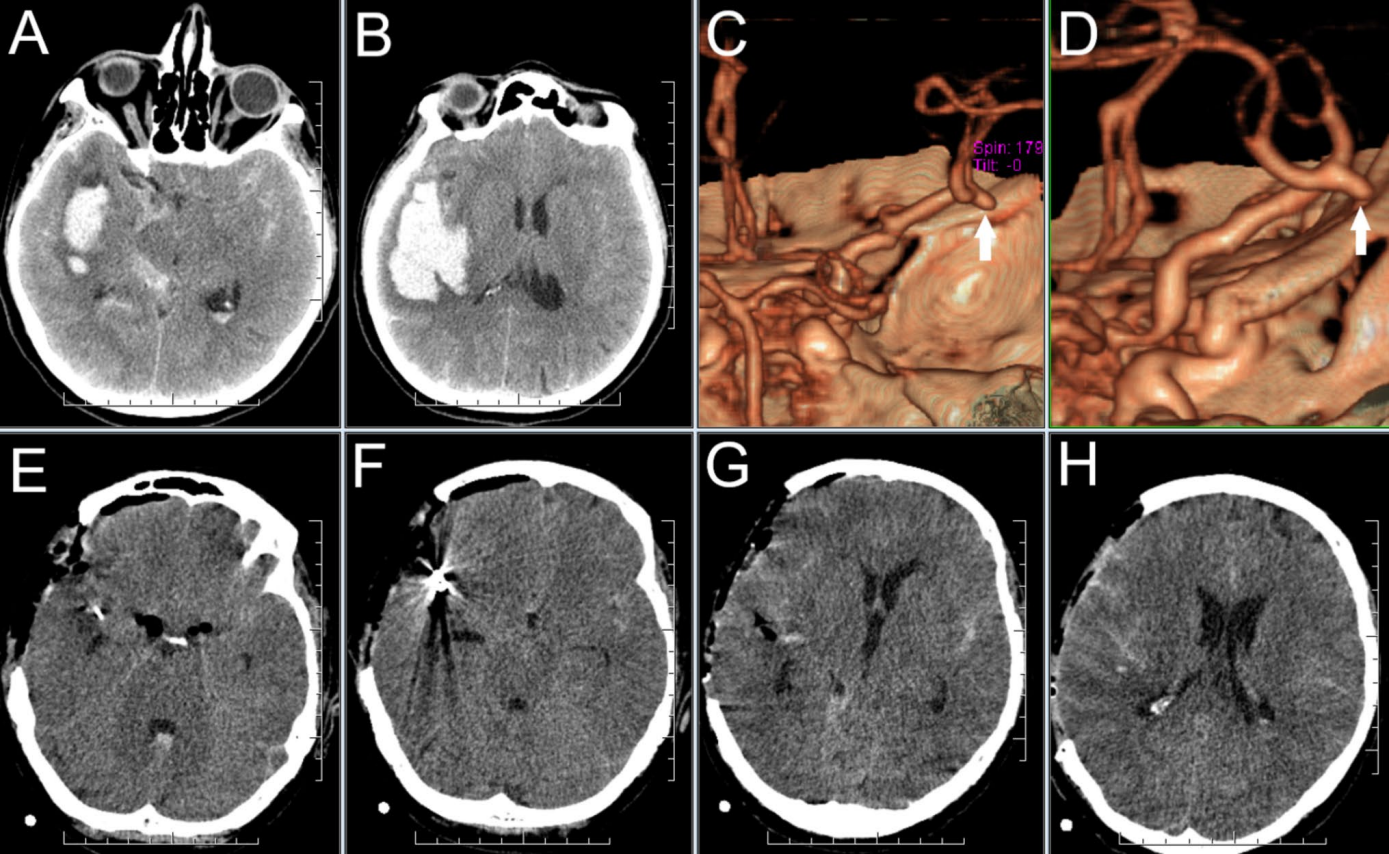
Ruptured right-sided MCA aneurysm – case No. 10 A and B: Preoperative CT showing massive subarachnoid hemorrhage and large right sided temporal hematoma extending into deeper brain structures. C and D: 3D reconstruction of CT angiography showing right-sided MCA aneurysm directed to the temporal lobe. E – H CT images performed on the first postoperative day
